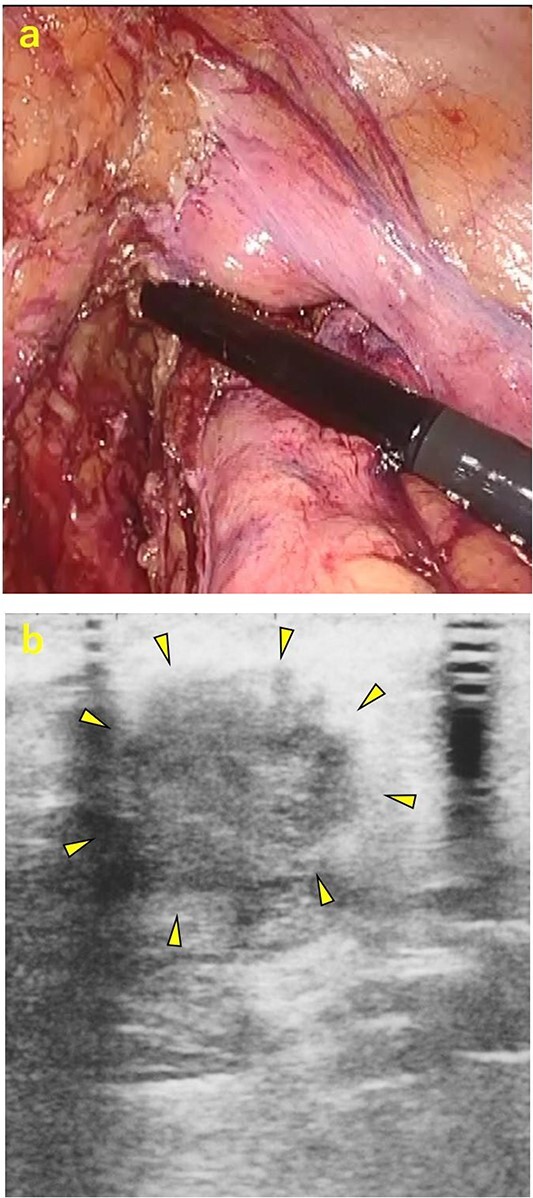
Intraoperative ultrasonography. (a) A laparoscopic ultrasonography probe was applied to the mesorectum to identify the mass. (b) A hypoechoic area of ~20 mm
